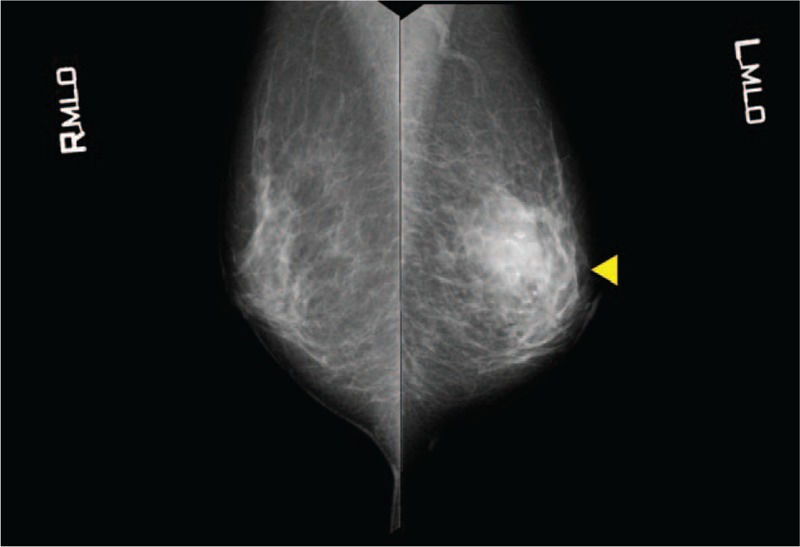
A hypoechoic area measuring 18 × 31 × 24 mm with partially calcified areas and a hyperechoic margin was observed in the C/D region of the left breast. The lesion had a Tsukuba elasticity score of 2 and internal blood flow and strongly suggested malignancy. A yellow arrowhead in the radiograph indicates the lesion.
